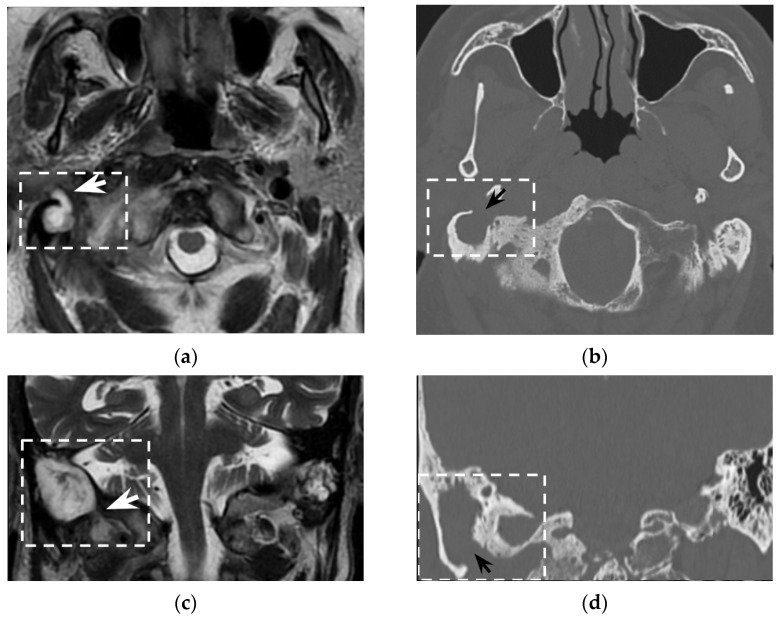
Eighty-seven-year-old man with history of external mycotic otitis. T2w MRI in axial (a) and coronal (c) planes show abnormal right mastoid (dotted rectangle) filled with hyperintense material that spreads into the neck (white arrows). Axial (b) and coronal (d) bone CT images show opacified right mastoid, absence of bone trabeculae, and a wide defect (black arrows) at the mastoid tip. Images were consistent with an infected cholesteatoma eroding the mastoid tip and spreading into the neck. The finding was confirmed at surgery.
